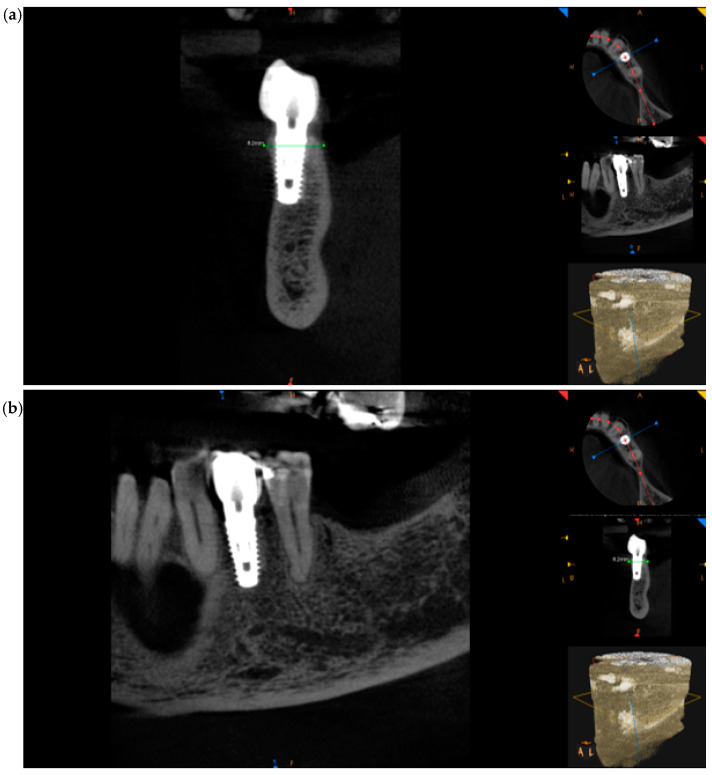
(a) CBCT pictures after a year (sagittal section) and (b) CBCT pictures after a year (coronal section).
